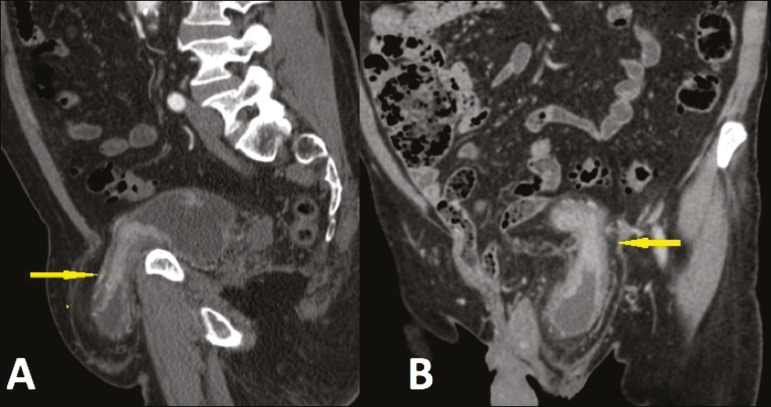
Sagittal and coronal CT scans (A and B, respectively) in the arterial phase, showing left inguinal herniation of the bladder. Note the area of irregular wall thickening and hypervascularization that proved to be a urothelial carcinoma.
